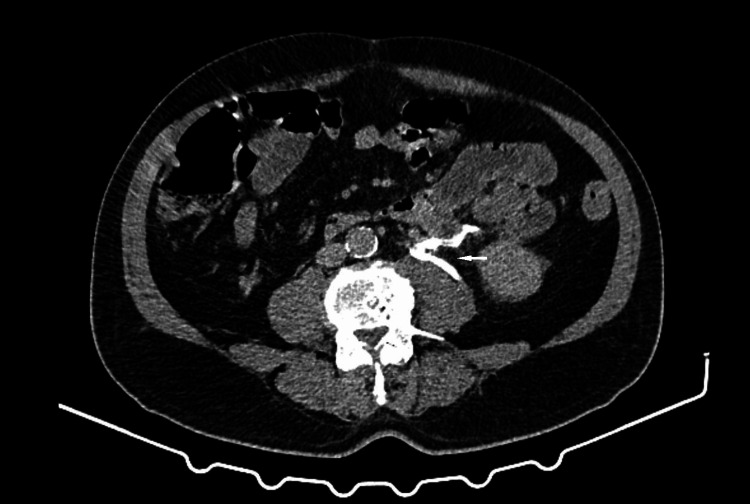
Axial CT image obtained after intravenous contrast administration demonstrating leakage of contrast material from the proximal left ureter, consistent with ureteral rupture.
